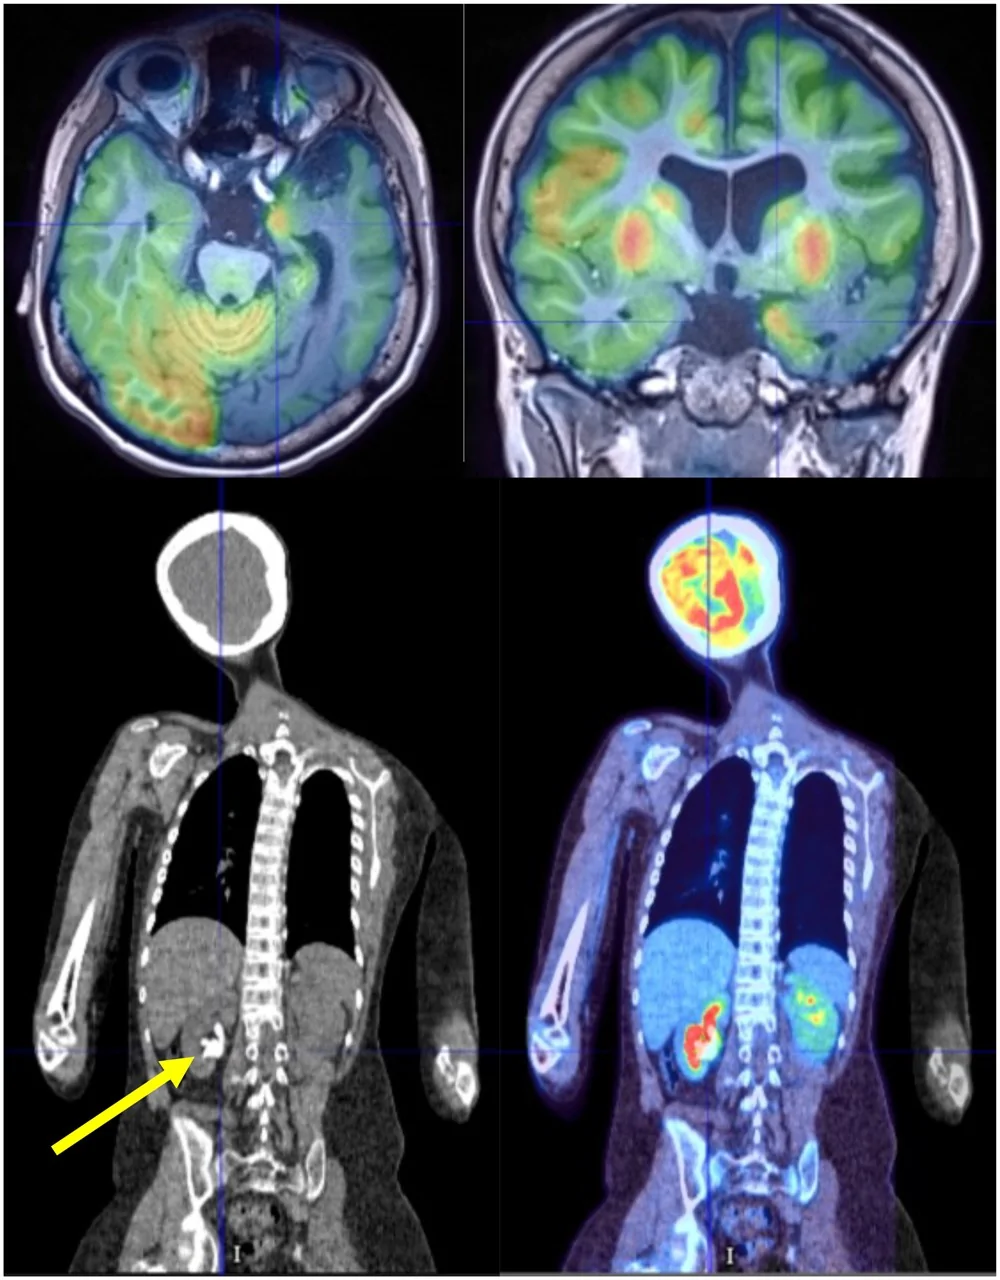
Example of useful cerebral and extracerebral information from large axial field-of-view PET—boy with learning disability assessed with [^18^F]FDG PET for epilepsy surgery. Brain PET coregistered to MRI identified the likely seizure onset zone (PET obtained 1 h postictally); body CT identified a staghorn calculus in the right kidney as a likely cause for unexplained recurrent hematuria.
